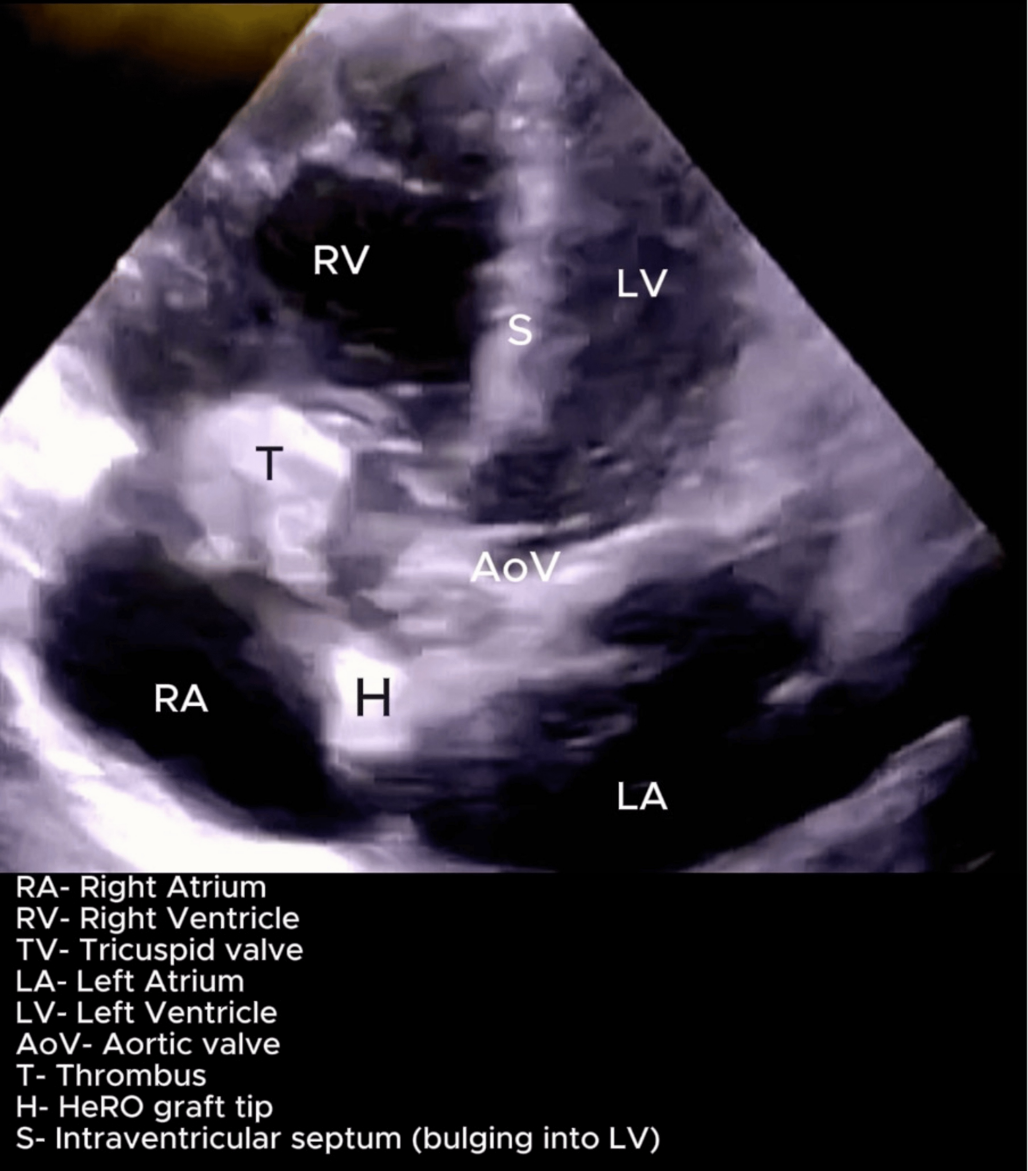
POCUS heart: Apical five-chamber view showing the HeRO graft tip and the thrombus attached to the tricuspid valve. Additionally, there are features of right ventricular strain with bulging of the interventricular septum into the left ventricle. Probe: Phased array probe. View: Apical five-chamber view. Depth: 18 cm. POCUS, point-of-care ultrasound; HeRO, Hemodialysis Reliable Outflow
